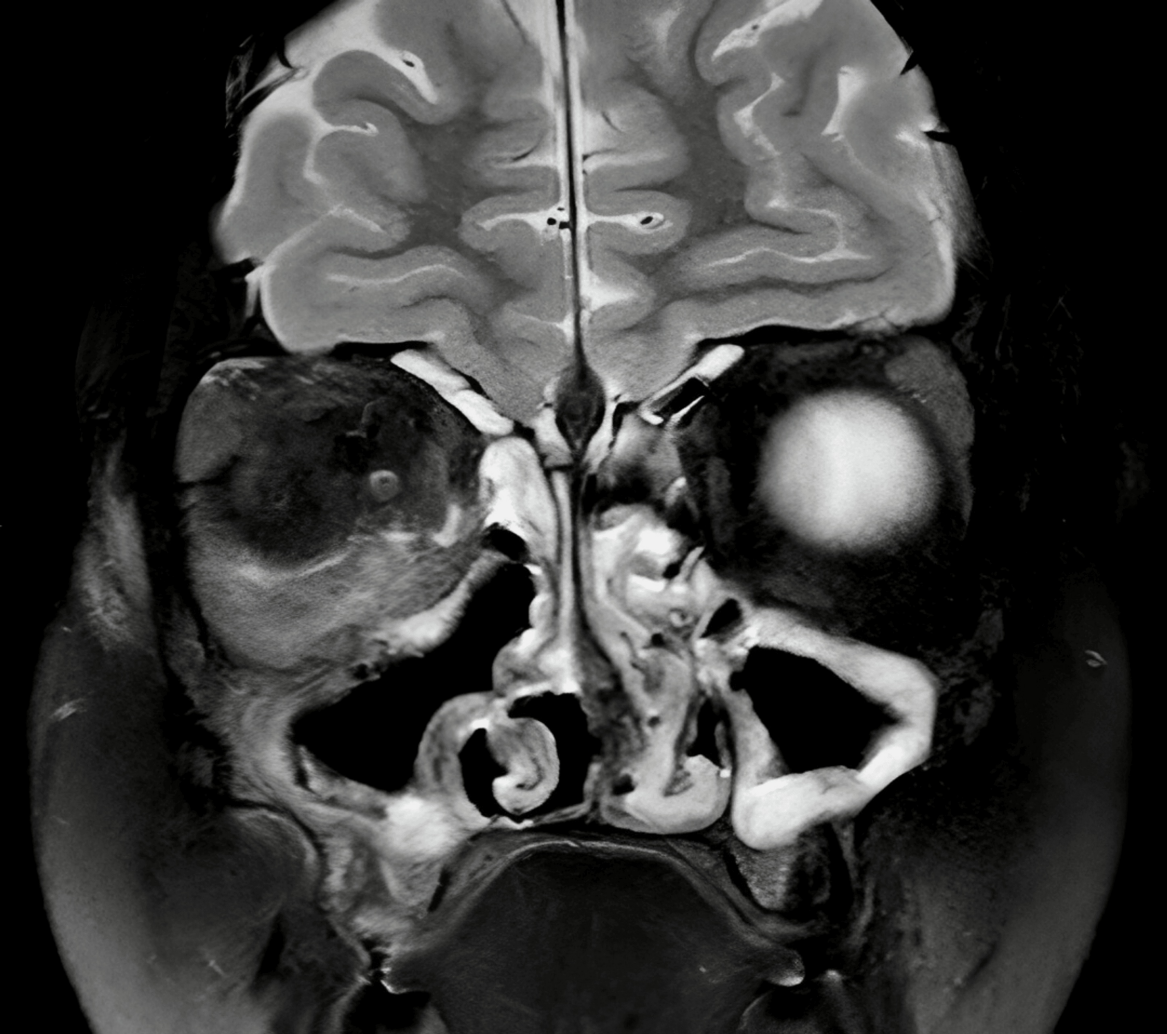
MRI Brain showing widespread inflammation around the right orbit and bilateral nasal sinuses
